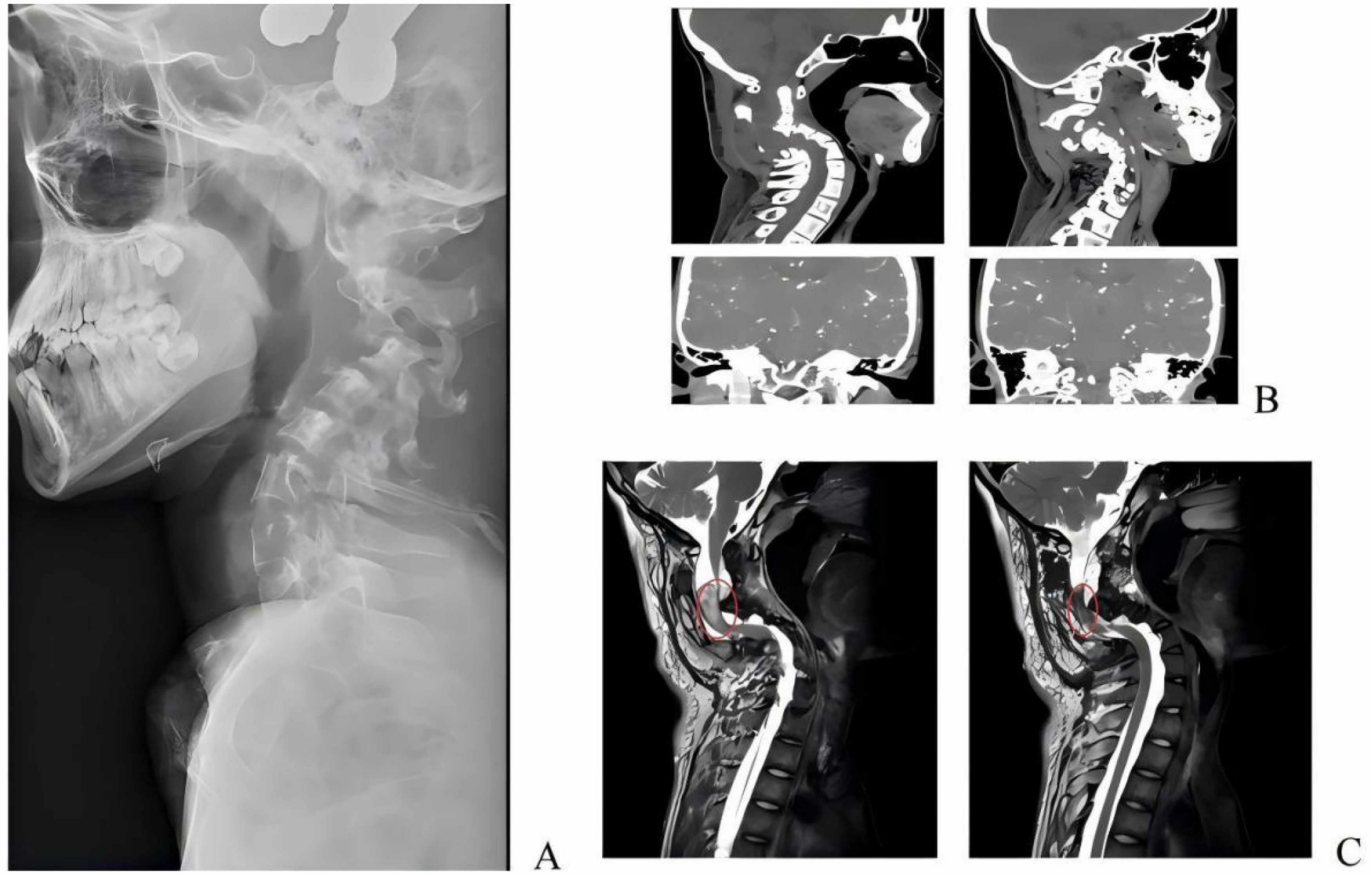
The patient's imaging findings included a cervical lateral radiograph (A), cervical computed tomography (CT; B), and cervical magnetic resonance imaging (MRI; C), where the tumor location was highlighted with a red circle in the corresponding images.
